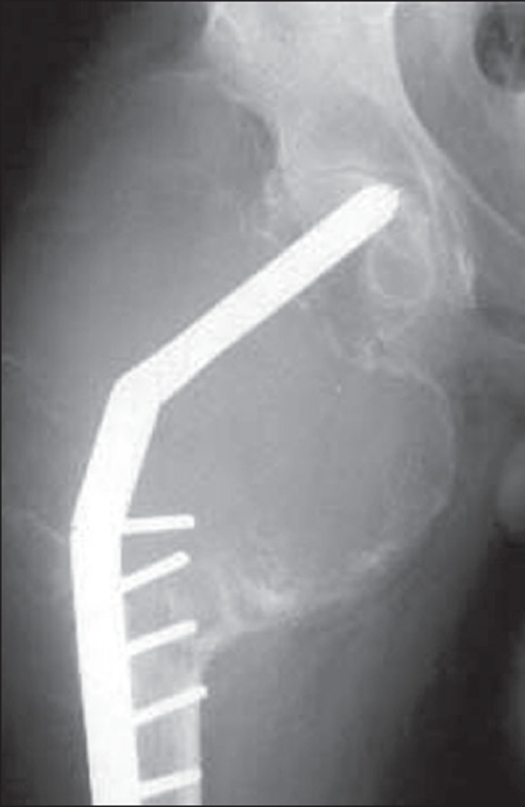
A case of recurrent GCT of proximal femur at 18 months follow up. The x-rays shows extensive cortical expansion with impending implant breakage
